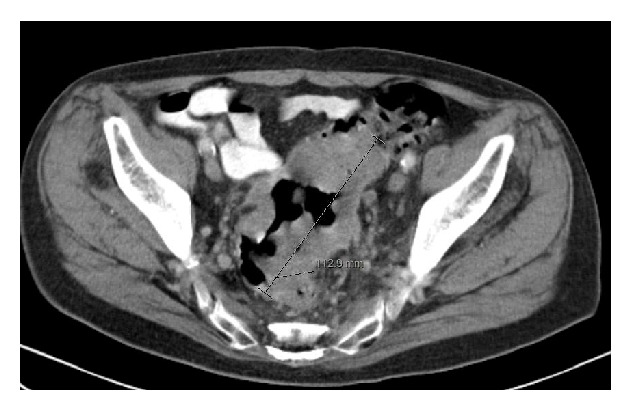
CT scan revealing 11.3 cm sigmoid mass.
